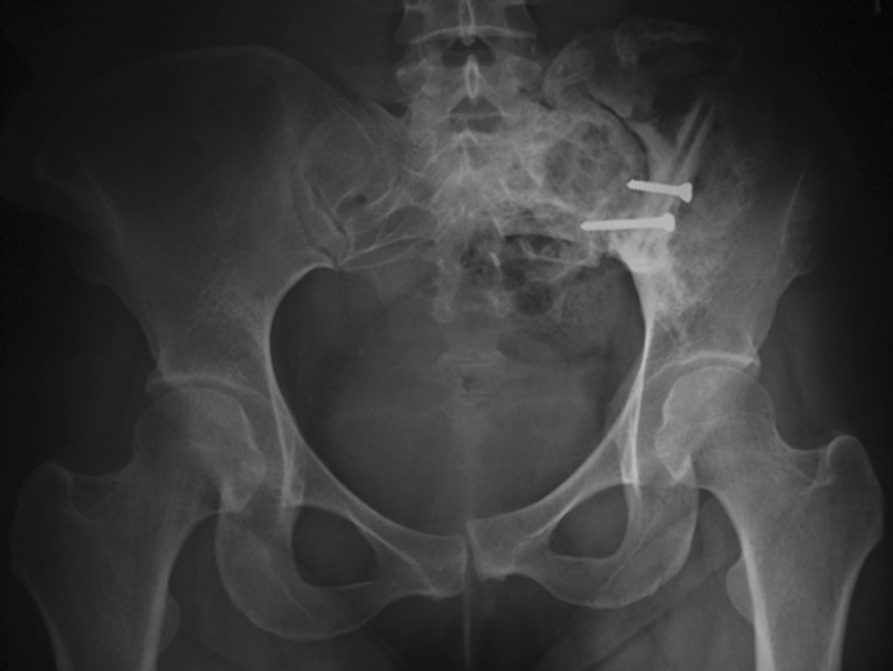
At the postoperative 9-year follow-up radiography shows slight displacement of the pubic symphysis and sacroiliac joint and good bone healing with no tumor recurrence.
